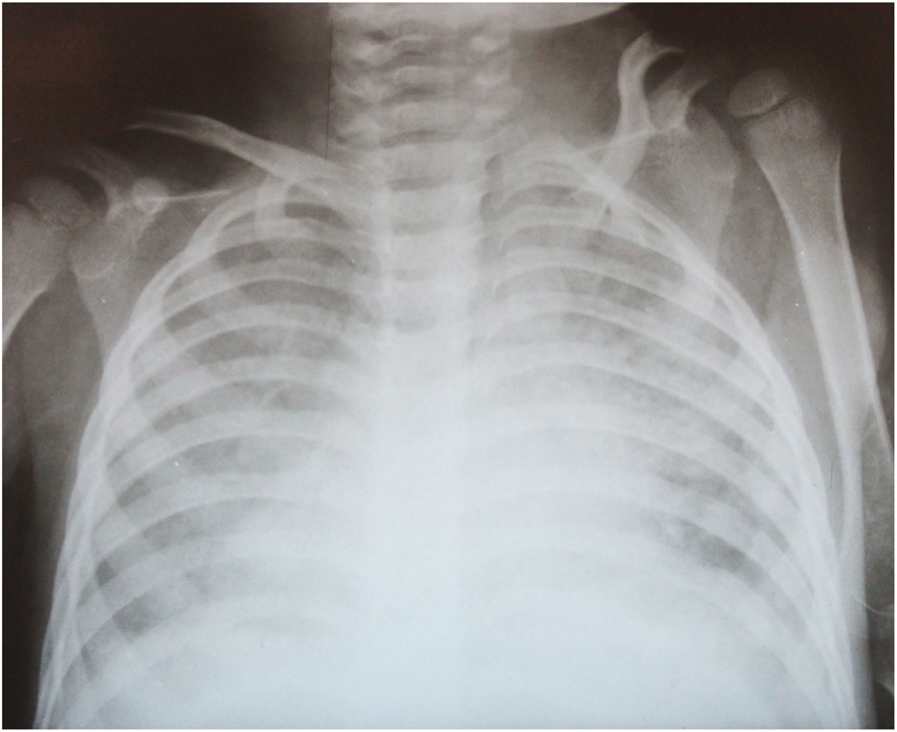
Chest X-ray just after extubation
